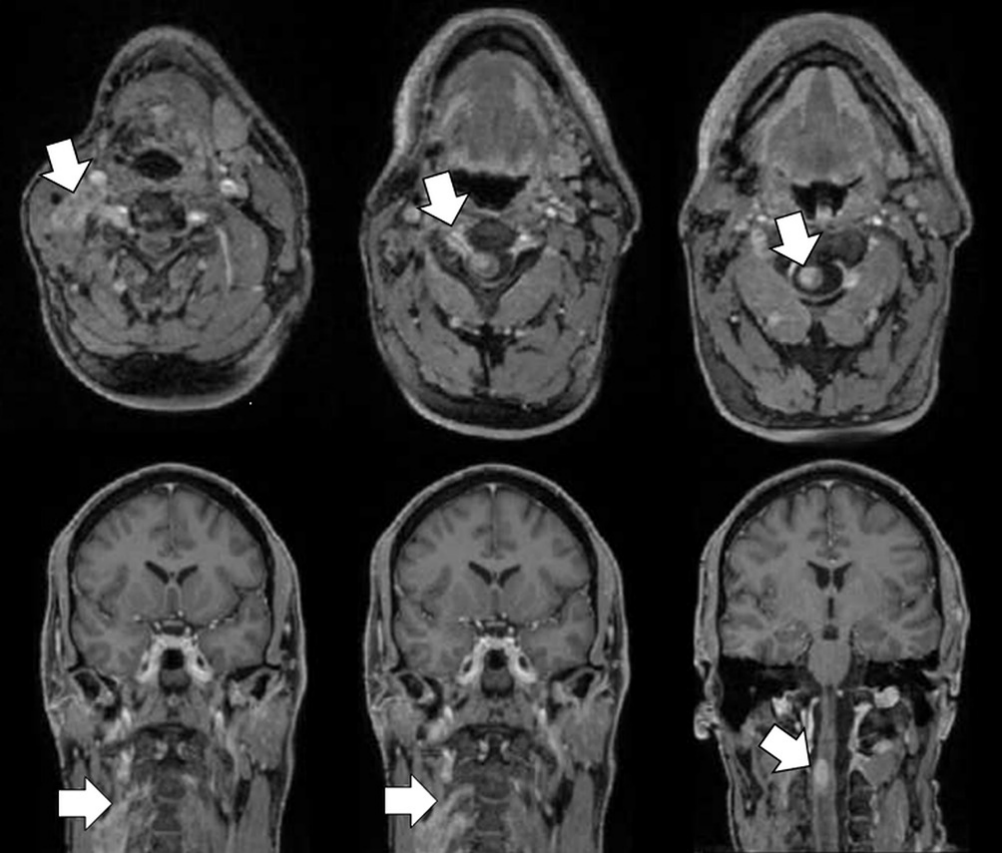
Post-gadolinium *T*_1_ fat-suppressed axial and coronal MR images demonstrate an enhancing mass in the right side of the neck representing local melanoma recurrence, with perineural spread along the right C3 and C4 nerves and contiguous invasion into the spinal cord (arrows).
